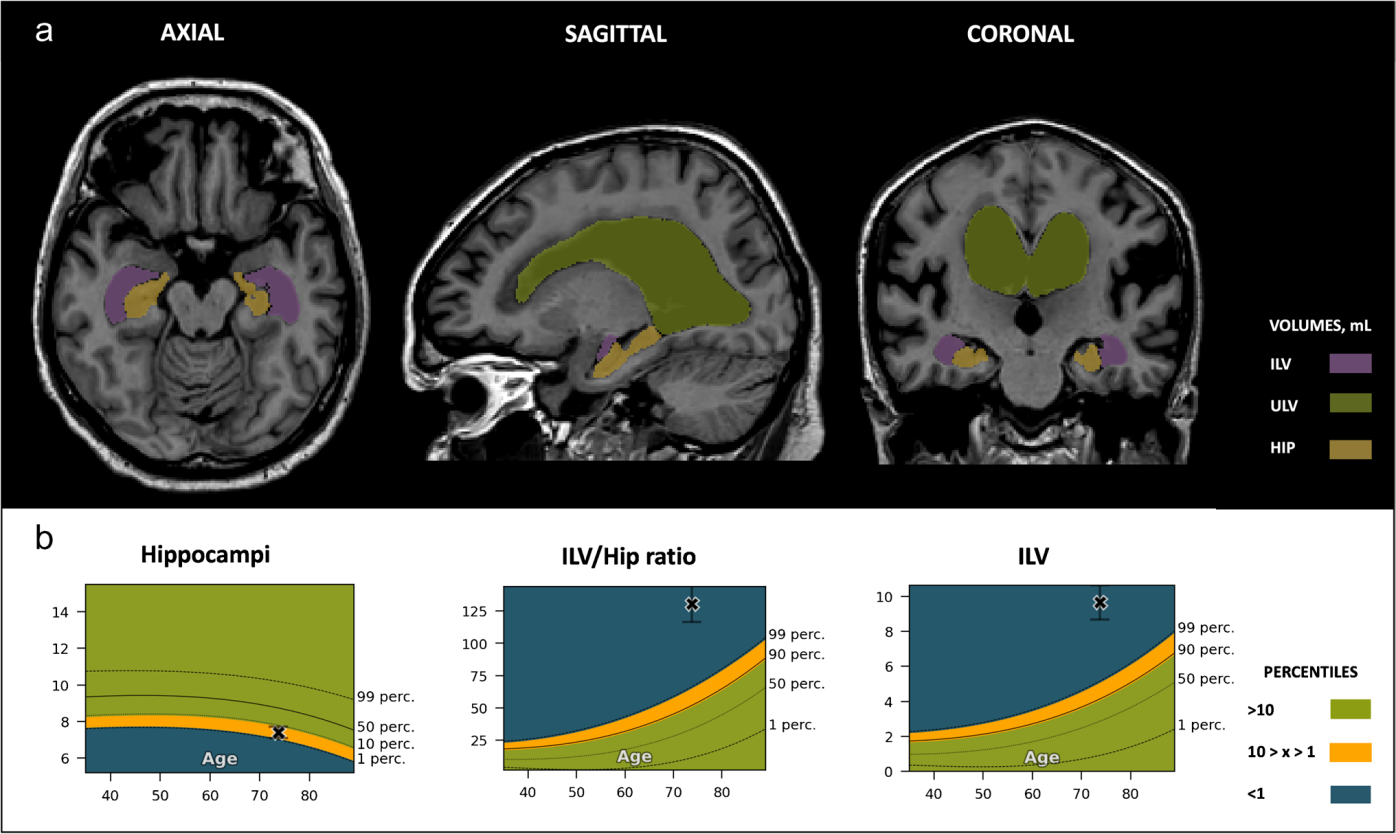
Normal-pressure hydrocephalus (NPH) case. Cross-sectional coronal T1-weighted image at the level of the medial temporal lobe and hippocampus with segmentation of the upper lateral ventricles (green), inferior lateral ventricle (purple), and hippocampus (yellow). A — Automated volumetric measurements *left*: axial view *middle*: sagittal view *right*: coronal view. B — Population graphs *left*: hippocampal volumes of this subject showing the gray “x” marker in the orange region, between the 1st and 10th percentile *middle*: the ILV/Hip ratio of this subject showing the gray “x” marker in the blue region, above the 99th percentile. *Right*: ILV volumes of this subject showing the gray “x” marker in the blue region, above the 99th percentile. Color-coded population graph percentile interpretation; 1–90th percentiles, green (normal); 90–99th percentiles, orange (caution); > 99th percentile, dark blue (abnormality)
